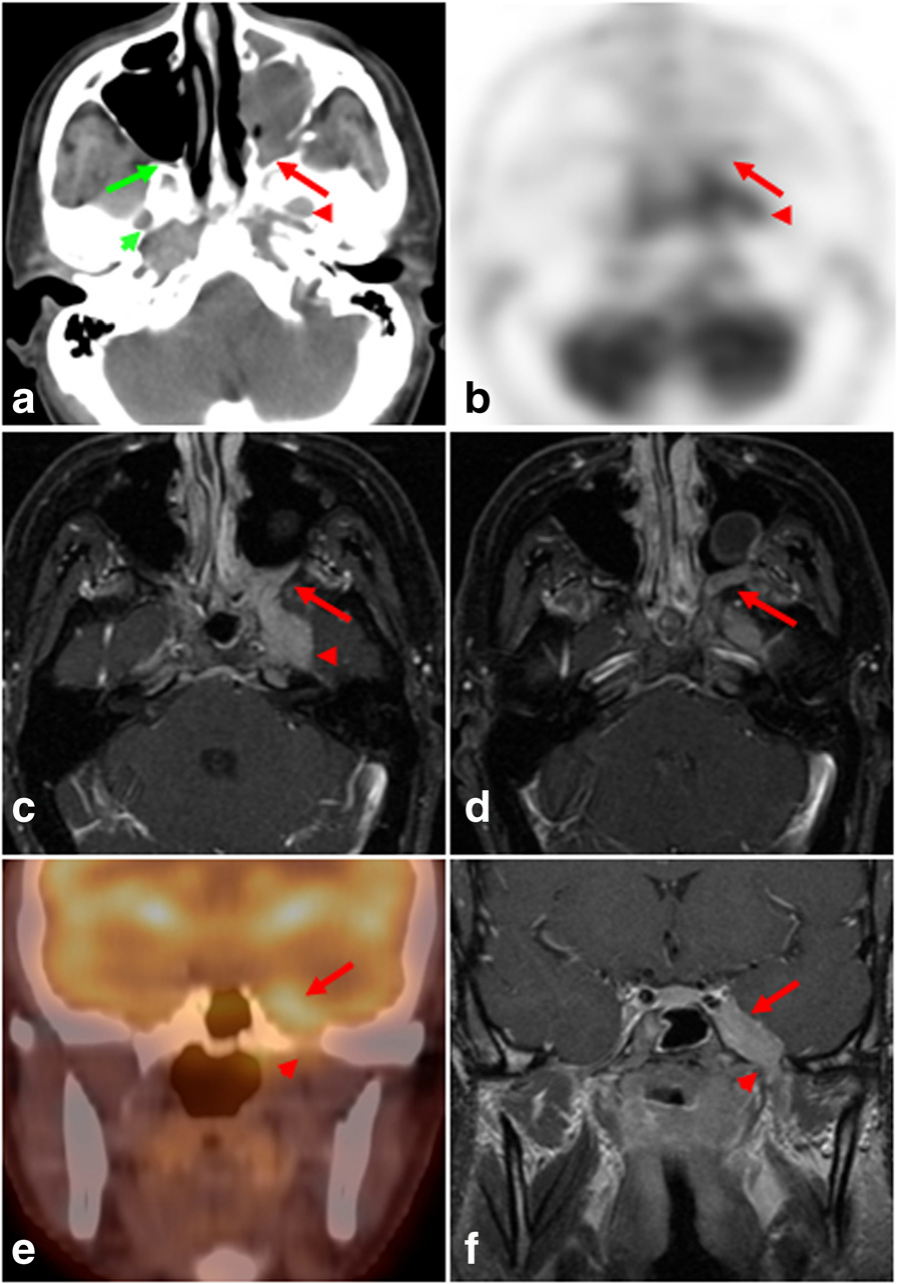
A 41-year-old male with nasopharyngeal squamous cell carcinoma. Axial CT (a), and PET (b) images show an iso/hyperdense hypermetbolic mass extending into the enlarged left pterygopalatine fossa (red arrow) and foramen ovale (red arrowhead), confirming perineural tumor spread along the maxillary (V2) and mandibular (V3) divisions of the trigeminal (CNV) nerve. Note the normal appearance of the right pterygopalatine fossa (green arrow) and foramen ovale (green arrowhead). Axial contrast-enhanced fat suppressed T1 SI MR (c, d) of the neck confirm extension of the enhancing soft tissue mass from the left cavernous sinus/Meckel’s cave region in to the left pterygopalatine fossa (red arrow). Coronal fused PET/CT (e) and contrast-enhanced fat suppressed T1 SI MR (f) confirming perineural extension of the tumor along V3, infiltrating the left cavernous sinus/ Meckel’s cave region (arrow) and widening the ipsilateral foramen ovale (arrowhead)
